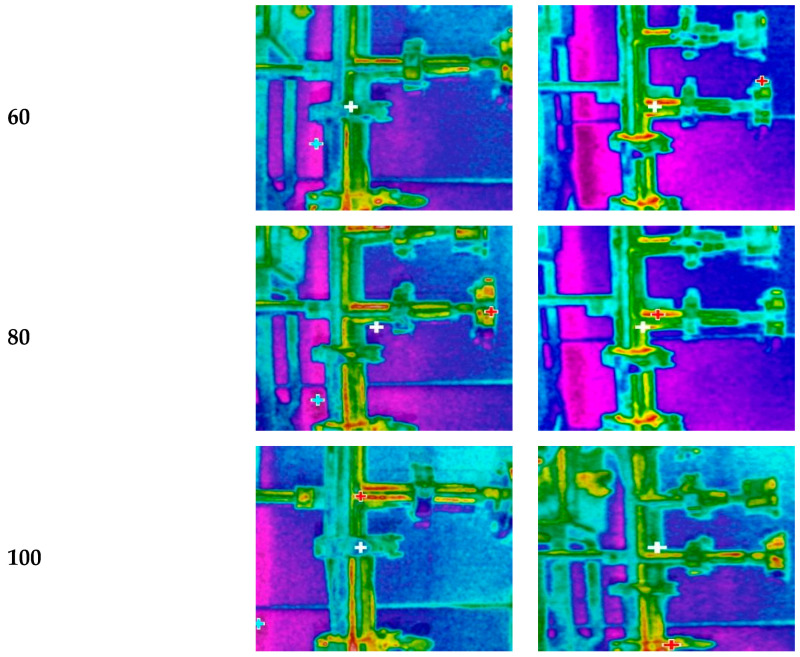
Thermal imaging of ultrasonic energy irradiation from two probes at different amplitudes of low-frequency ultrasound (LFUS) (without membrane filtration, U = 6 m·s^−1^).
